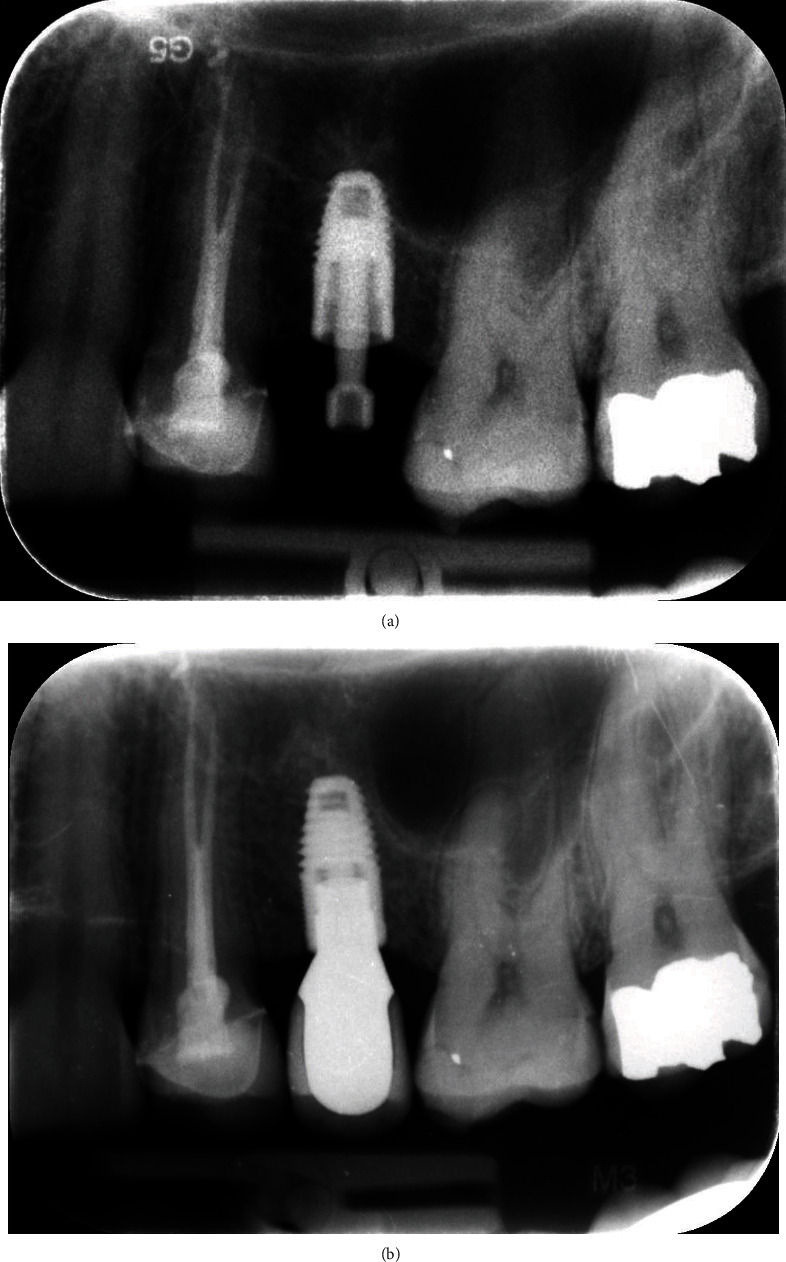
(a) Postoperatory endoral radiography. (b) Endoral radiography taken after 9 months from the surgery.
